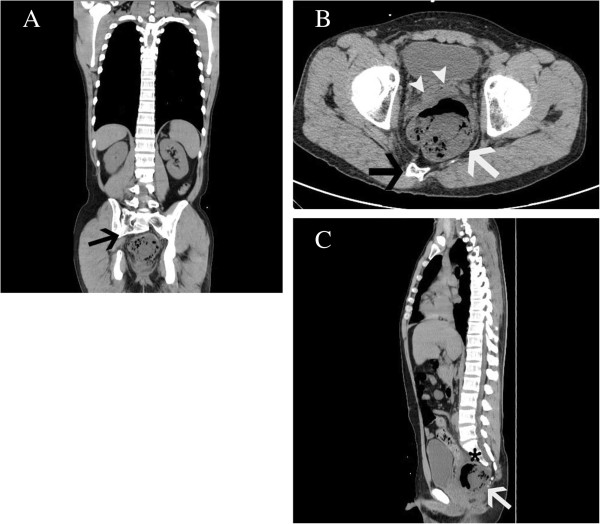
Computed tomography imaging findings. (A) A scimitar-shaped deformity of the sacrum (black arrow). (B) An 8cm presacral mass (white arrow) containing air displaced the rectum (white arrow heads) ventrally. (C) The mass appeared to continue to the spinal canal through the anterior wall of the sacrum (asterisk).
